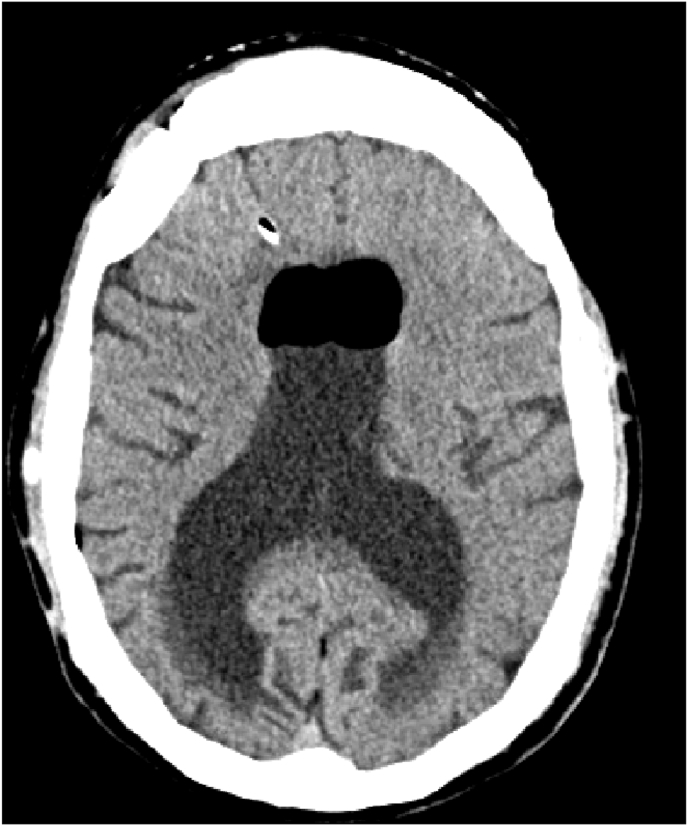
CT scan revealed the persistence of hydrocephalus and pnemocephalus
